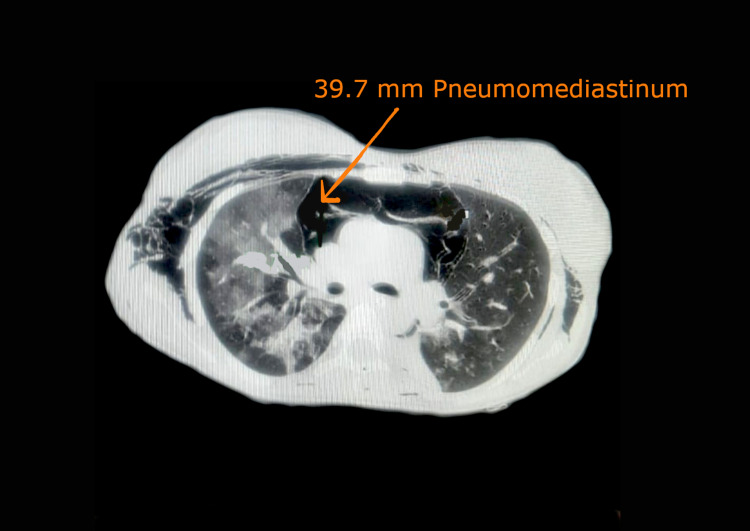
High-resolution computed tomography of the thorax shows bilateral pneumothorax with left parasternal 39.7 mm pneumomediastinum (Case 2)
